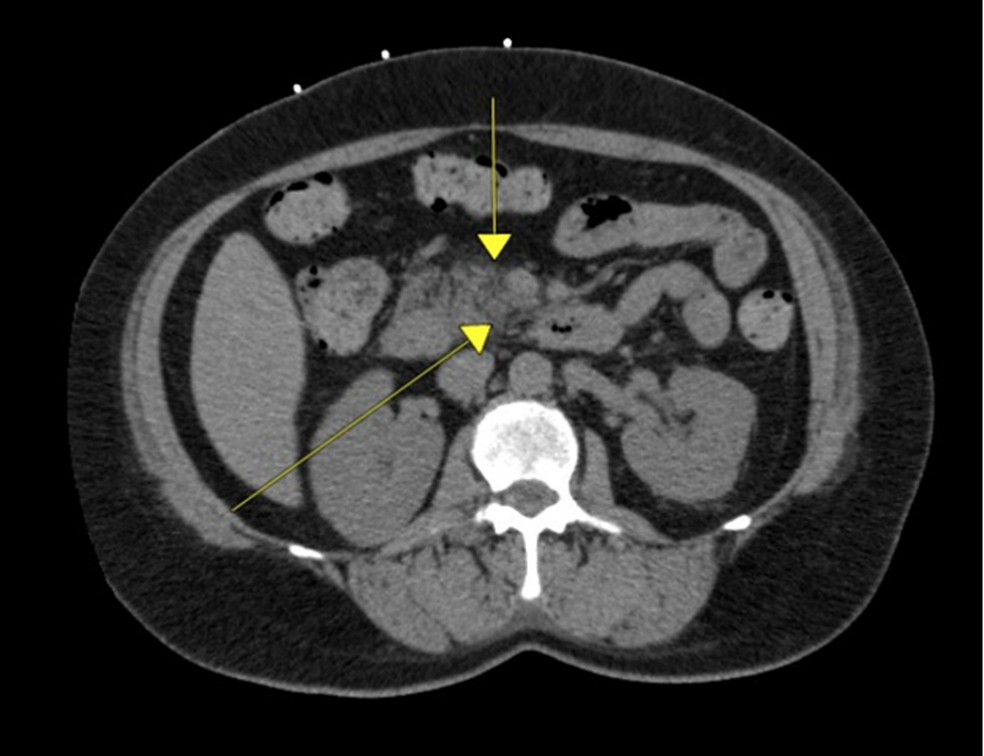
Computed tomography of abdomen and pelvis without contrast showing infiltrative/inflammatory change of fat around the pancreas.
